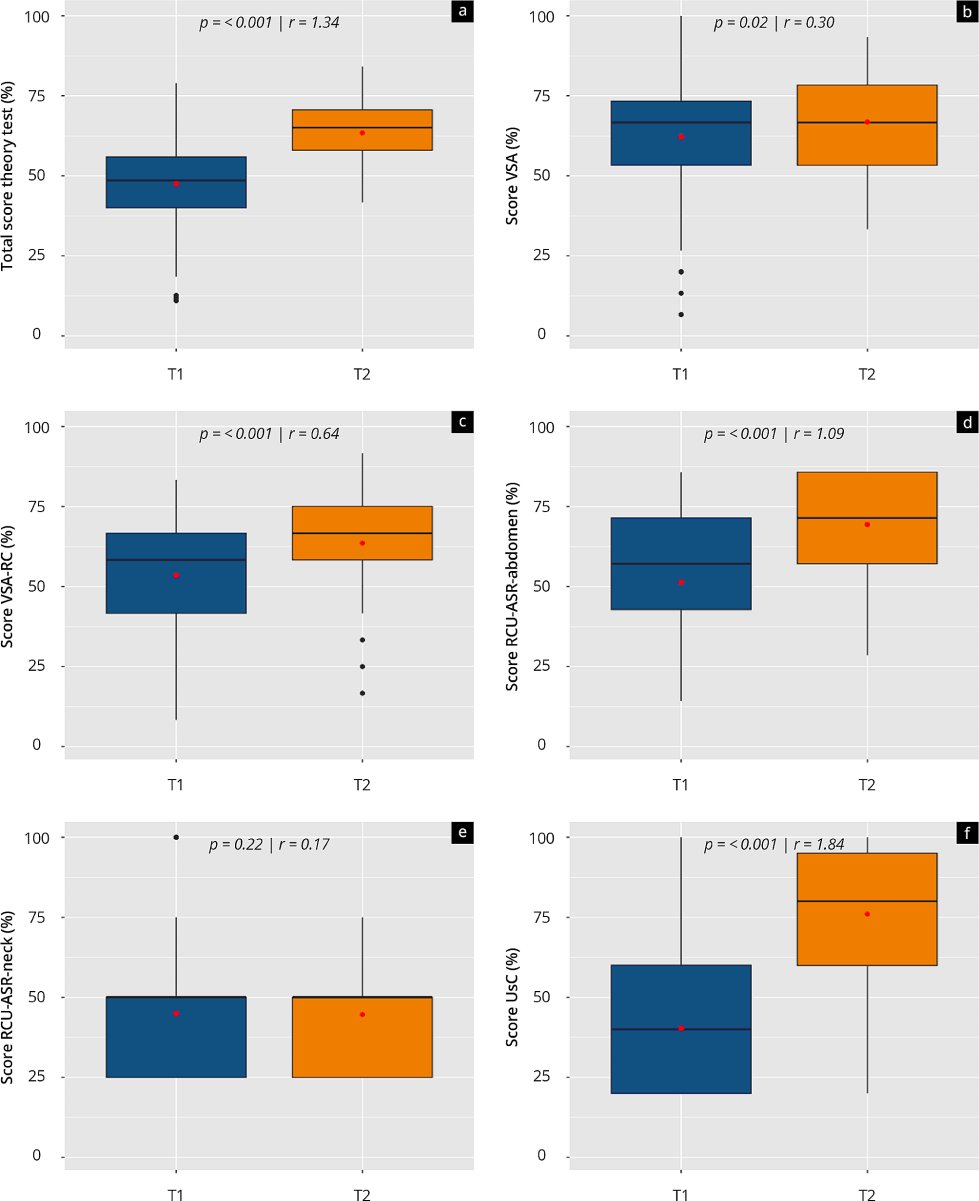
Results of the theory tests at time points T1(Theory_pre_) and T2 (Theory_post_). Box plots visualizing the respective overall score (a) as well as the score of the competencies: “VSA: visual-spatial ability” (b), “VSA-RC: Visual-spatial ability in radiological cross-sectional images” (c), “RCU-ASR- abd: Understanding of radiological cross-sectional images and knowledge of anatomical spatial relationships in CT and MRI images in the abdomen” (d), “RCU-ASR-neck: Understanding of radiological cross-sectional images and knowledge of anatomical spatial relationships in CT and MRI images in the neck” (e), and “UsC: theoretical ultrasound competences” (f). A high number implies a high percentage performance in the test. The median (black lines), mean (red dots), and the effect size r are shown
